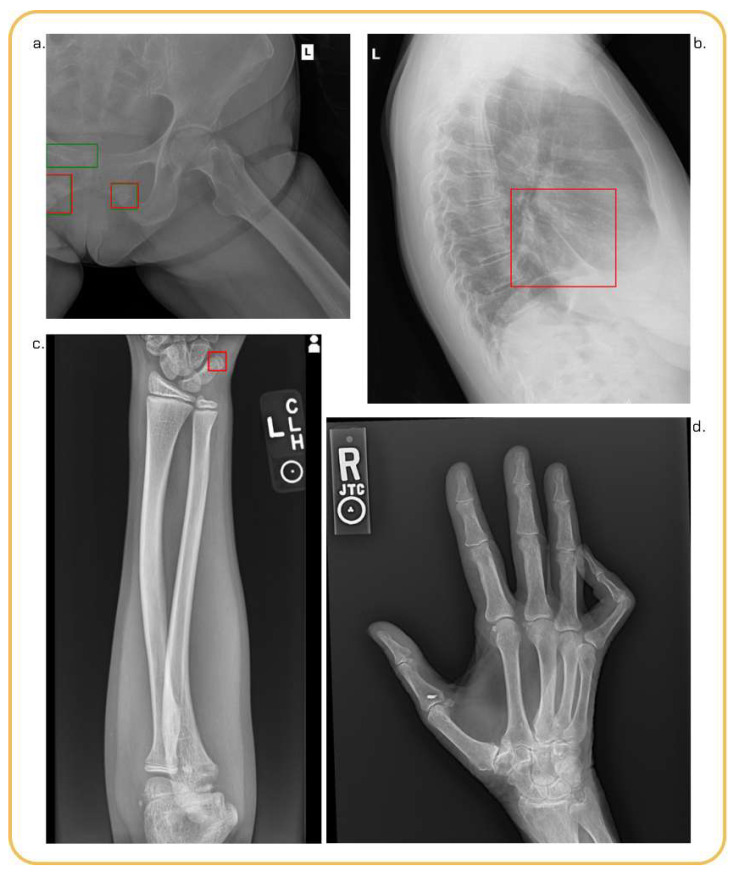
Examples of standalone performance of the Rayvolve^®^ AI suite. Green and red boxes respectively represent GT and the AI model predictions. (a) AZtrauma correctly identified 2 fractures (TP) and missed the ilio pubic ramus fracture (FN). (b) AZchest incorrectly detected pleural effusion on the lateral view of lung (FP). (c) AZtrauma incorrectly detected a fracture on the right hand (FP). (d) AZtrauma correctly identified a normal radiography (TN).
